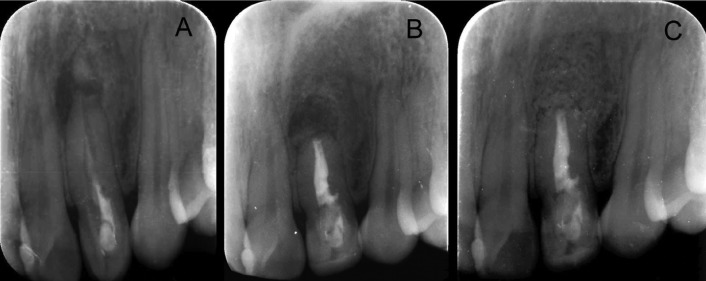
Preoperative periapical radiograph of tooth 2.2 showing previous endodontic treatment and the apical fracture (arrow) (A), post-surgery periapical radiography (B) and check periapical radiography follow-up at 18 months after treatment. Complete periradicular healing (C).
